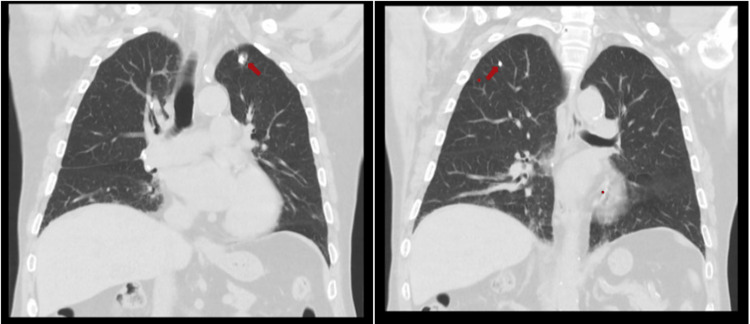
Chest CT. Red arrows show some of the granulomas. CT scan reassessment after beginning steroids did not show modifications.
